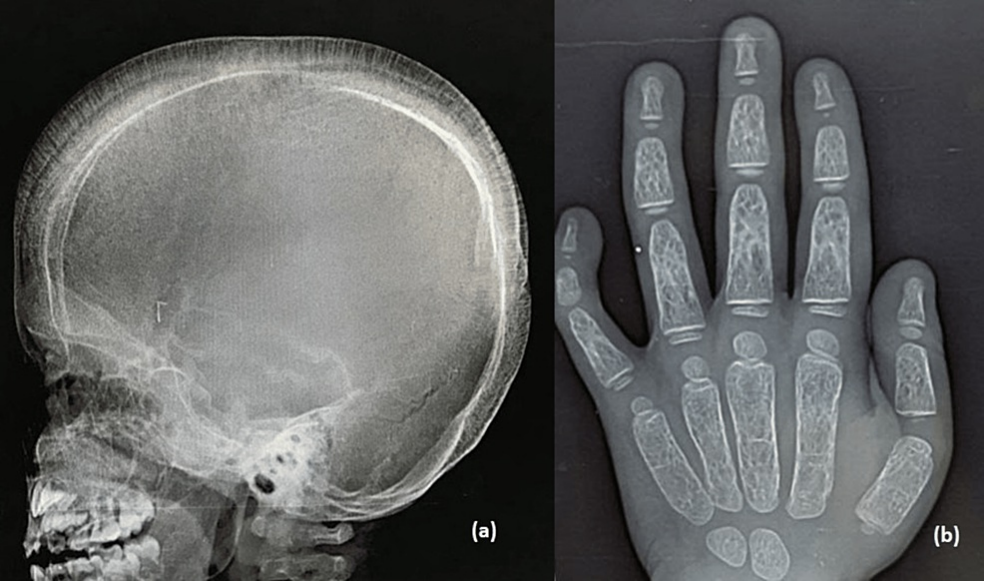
Lateral skull radiograph (a) showing widened diploic space with “hair-on-end” appearance and thinning of the outer table of the skull. Sparing of the occipital bone is seen (due to lack of hematopoietic bone marrow). AP hand radiograph (b) showing osteopenia, sausage-shaped tubular bones with cortical thinning, medullary expansion, and coarse trabeculation.
